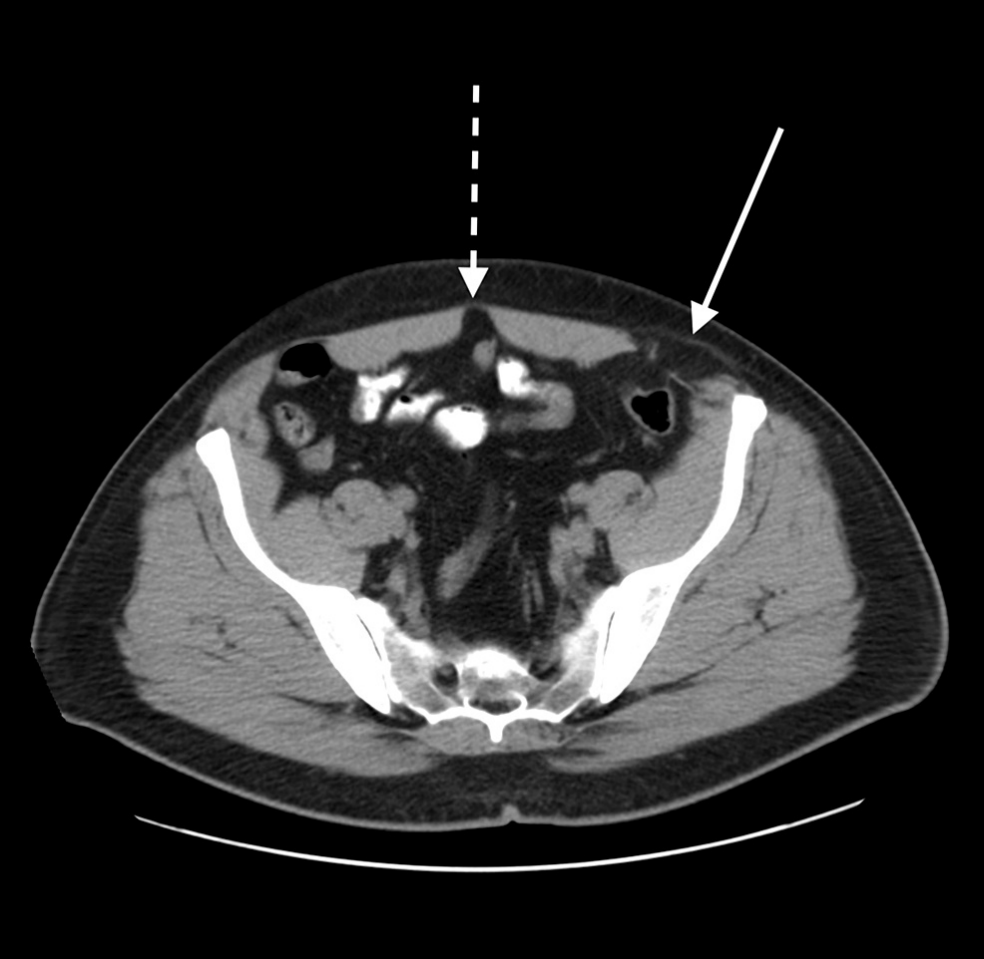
CT scan of the abdomen and pelvis without contrast, which displays a periumbilical incisional hernia (dotted arrow) and a left-sided Spigelian hernia (plain arrow).
